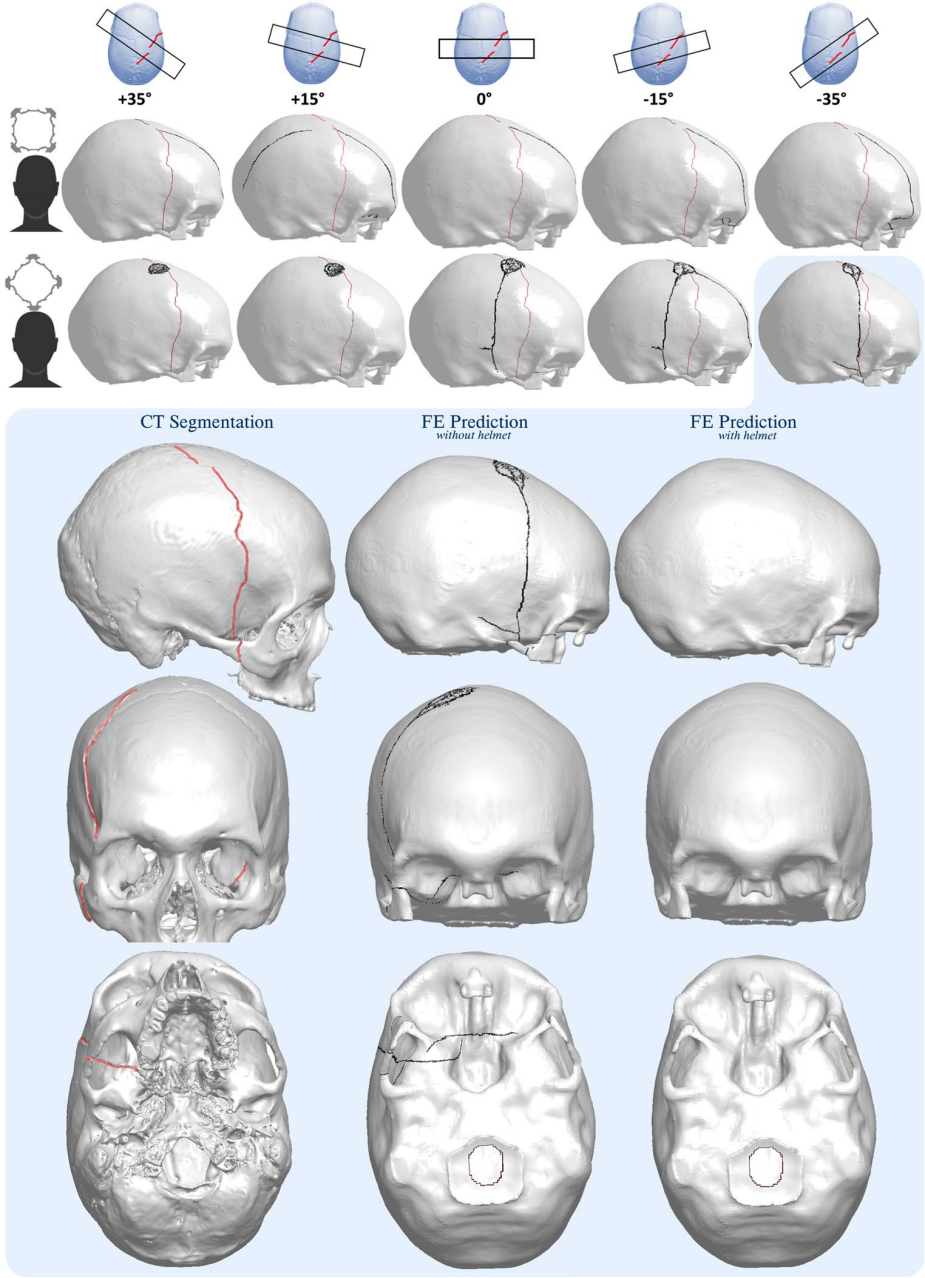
Predicted skull fractures in a selection of impact modes, altering the impact angle of the jack post and its impacting surface. The red fracture line represents the fracture segmented from CT, while the black represents the through-thickness-fracture predicted by the FE model. The impact scenario that predicted the fracture similar to the fracture of the victim is presented in detail
